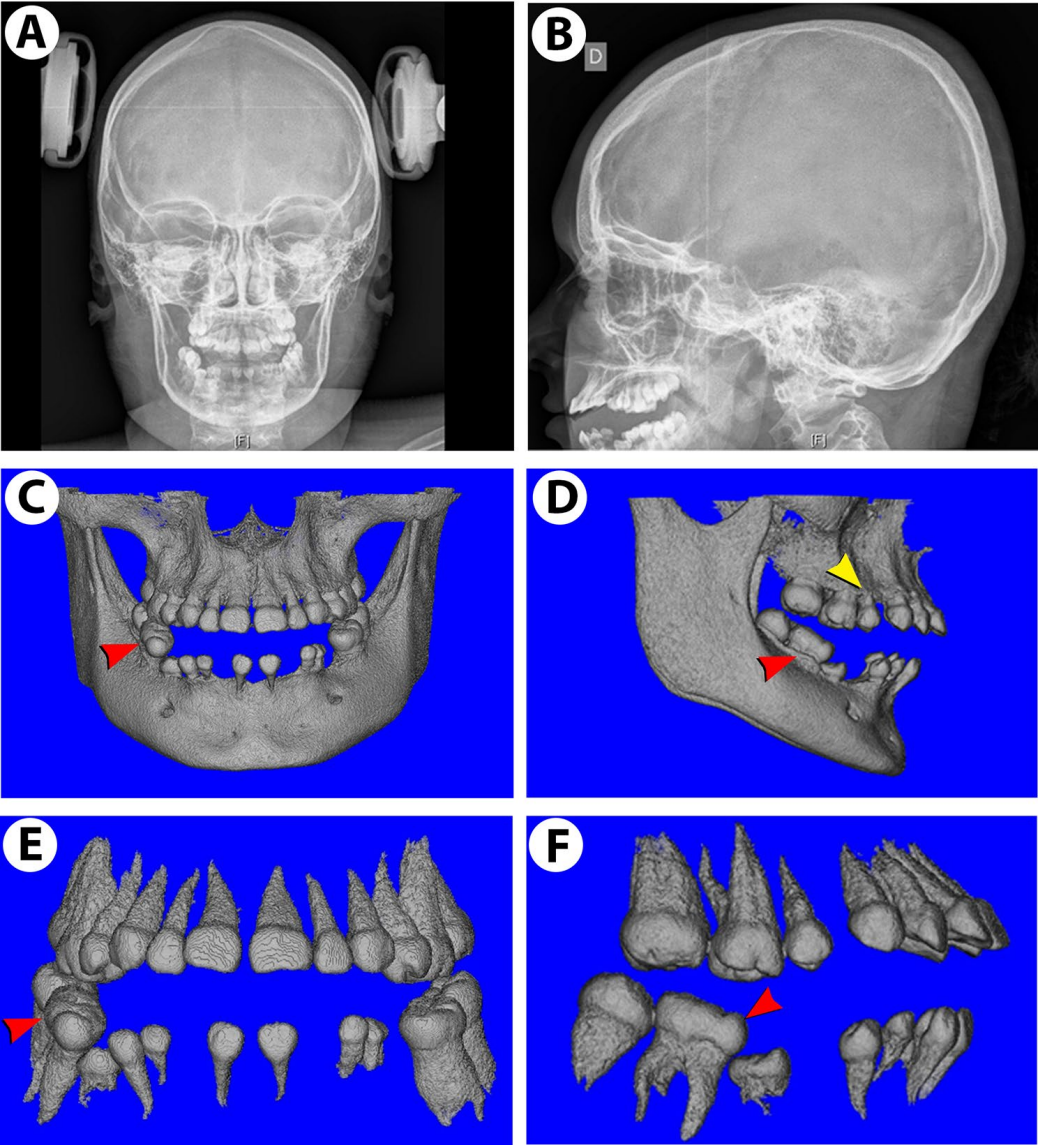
Radiographic and cone-beam computed tomography (CBCT) images of a 9 year-old girl with a homozygous *SMOC2* mutation (also see Ref.^12^). (A,B) Respective frontal and lateral radiographic views of the skull. Cranial bones and sutures, sella turcica, and orbit structures are normal except for the maxilla and mandible. (C) A full-frontal view of the dentition. The lower dentition displays more severe oligodontia and microdontia compared with the upper teeth. Affected teeth are typically 15–20% smaller than normal, except for the lower right first molar (red arrowhead), which is bigger. (D) Extracted CBCT images of full-lateral views. Defects include macrodontia (red arrowhead) and microdontia (yellow arrowhead). (E,F) Extracted frontal and lateral views with the lower dentition displaying more severe oligodontia and microdontia compared with the upper teeth. Image extraction of isolated teeth (using the Analyze 11.0 software) shows that a micro-root structure consistently accompanies microdontia. A false blue background was added using the Analyze 11.0 software (C–F), and the figure was labelled with Adobe Photoshop CS6.
